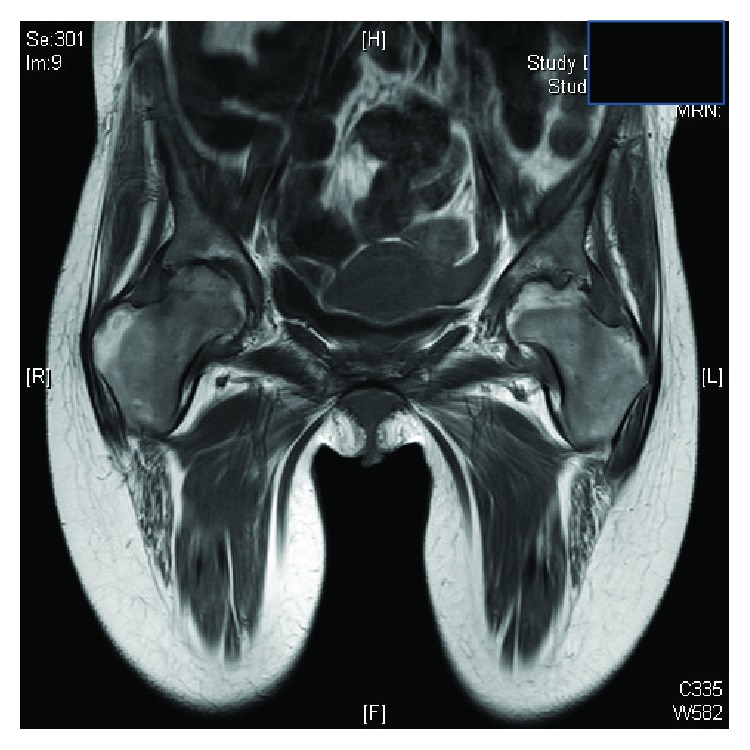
MRI of the hips at age 20.
